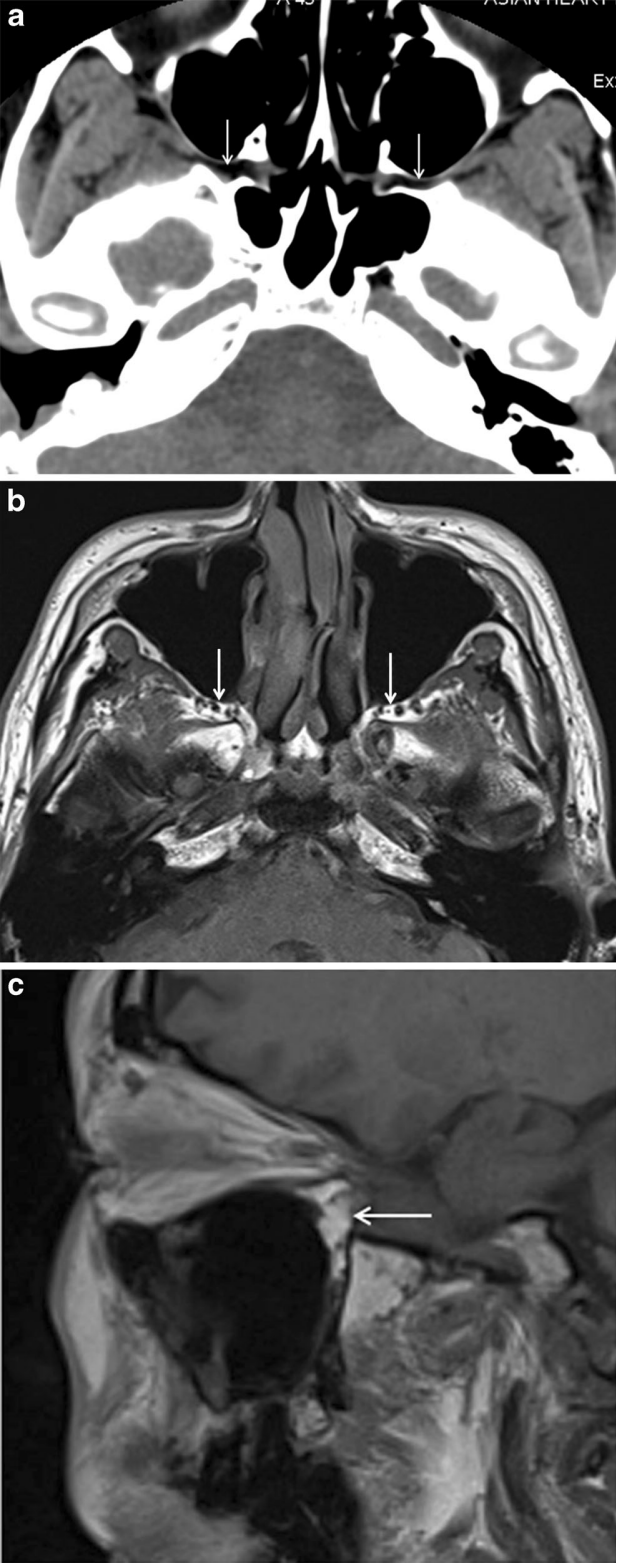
a Axial NECT image (soft-tissue window) showing normal hypodense appearance of bilateral fat-filled PPF (*arrows*). b and c Axial T1W MR image and sagittal T1W MR image showing hyperintense appearance of normal fat-filled PPF (*arrows*)
